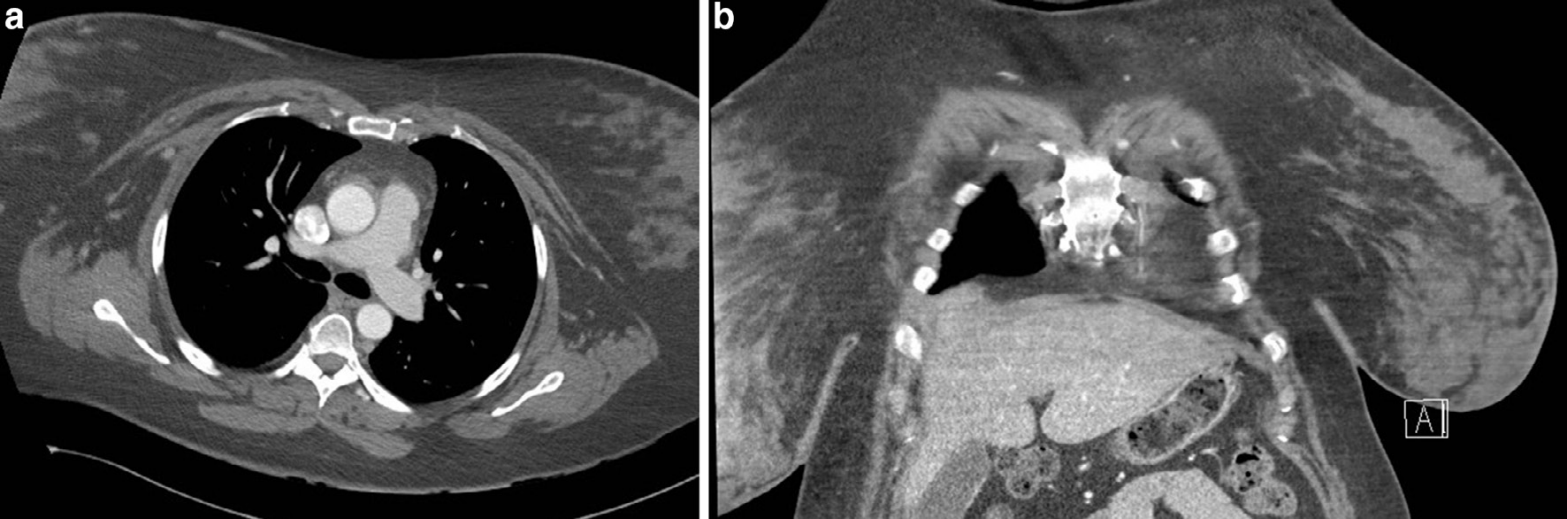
(a,b): Axial (a) and coronal (b) images of the thoracic CT angiogram demonstrating large breasts, more so on the right, partially included in the field of view.
